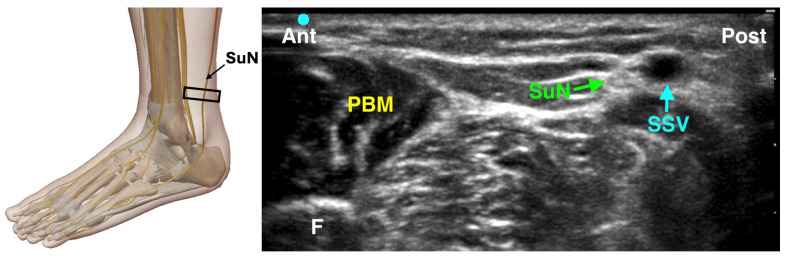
Ultrasound-guided Sural Nerve Block. SuN: Sural Nerve; SSV: Small Saphenous Nerve; PBM: Peroneus Brevis Muscle; F: Fibula; The black rectangle indicates the placement of the ultrasound probe on the anatomical area.
